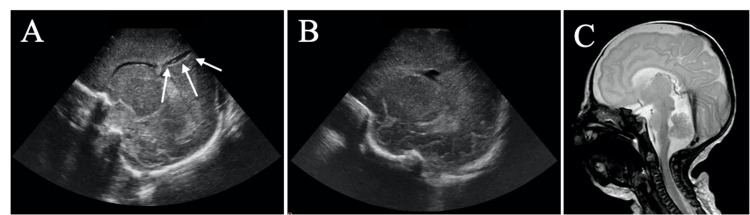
(A) Cerebral sonography of the newborn after difficult endotracheal intubation through the left nostril displaying a linear traumatic defect (white arrows) caused by the malpositioned nasotracheal tube. (B) Cerebral sonography and (C) MRI control with sagittal T2-weighted sequences after approximately three months show closure of the skull base defect. MRI: magnetic resonance imaging.
